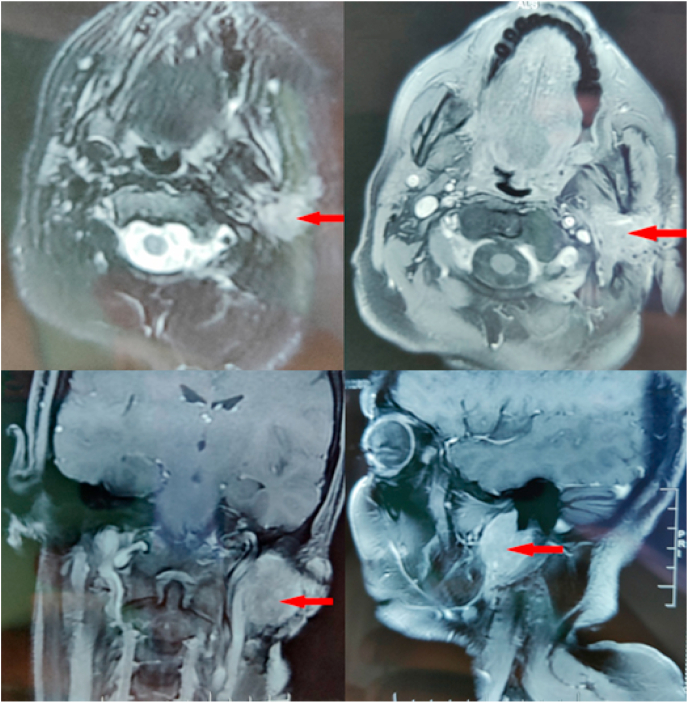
Facial MRI showing a left parotid tumor in isosignal T1 and T2.
